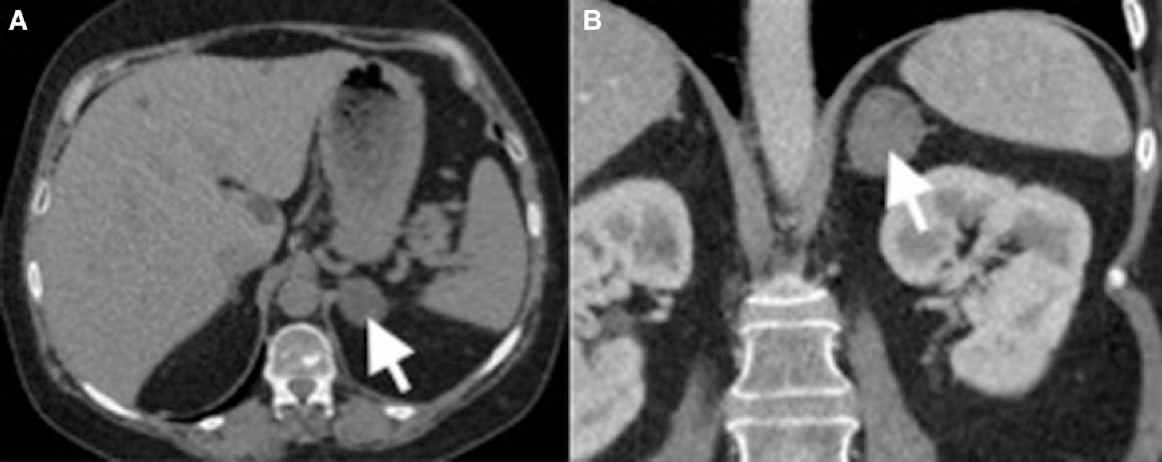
Computed tomography of the abdomen showing a 3.1-cm, lipid-rich adrenal adenoma (5 Hounsfield units). A, Axial view. B, Coronal view.
